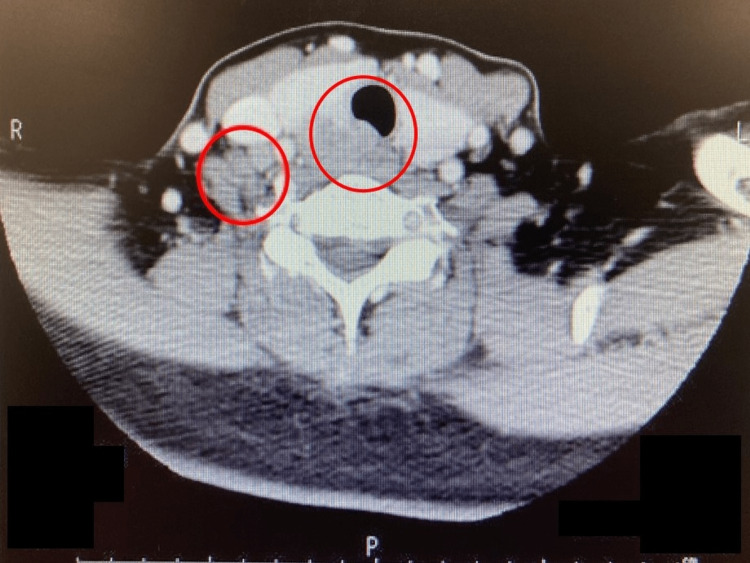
Plain chest computed tomography indicating a 31-mm mass in the medial side of the right lobe of the thyroid gland, and metastatic involvement of the right lymph node
